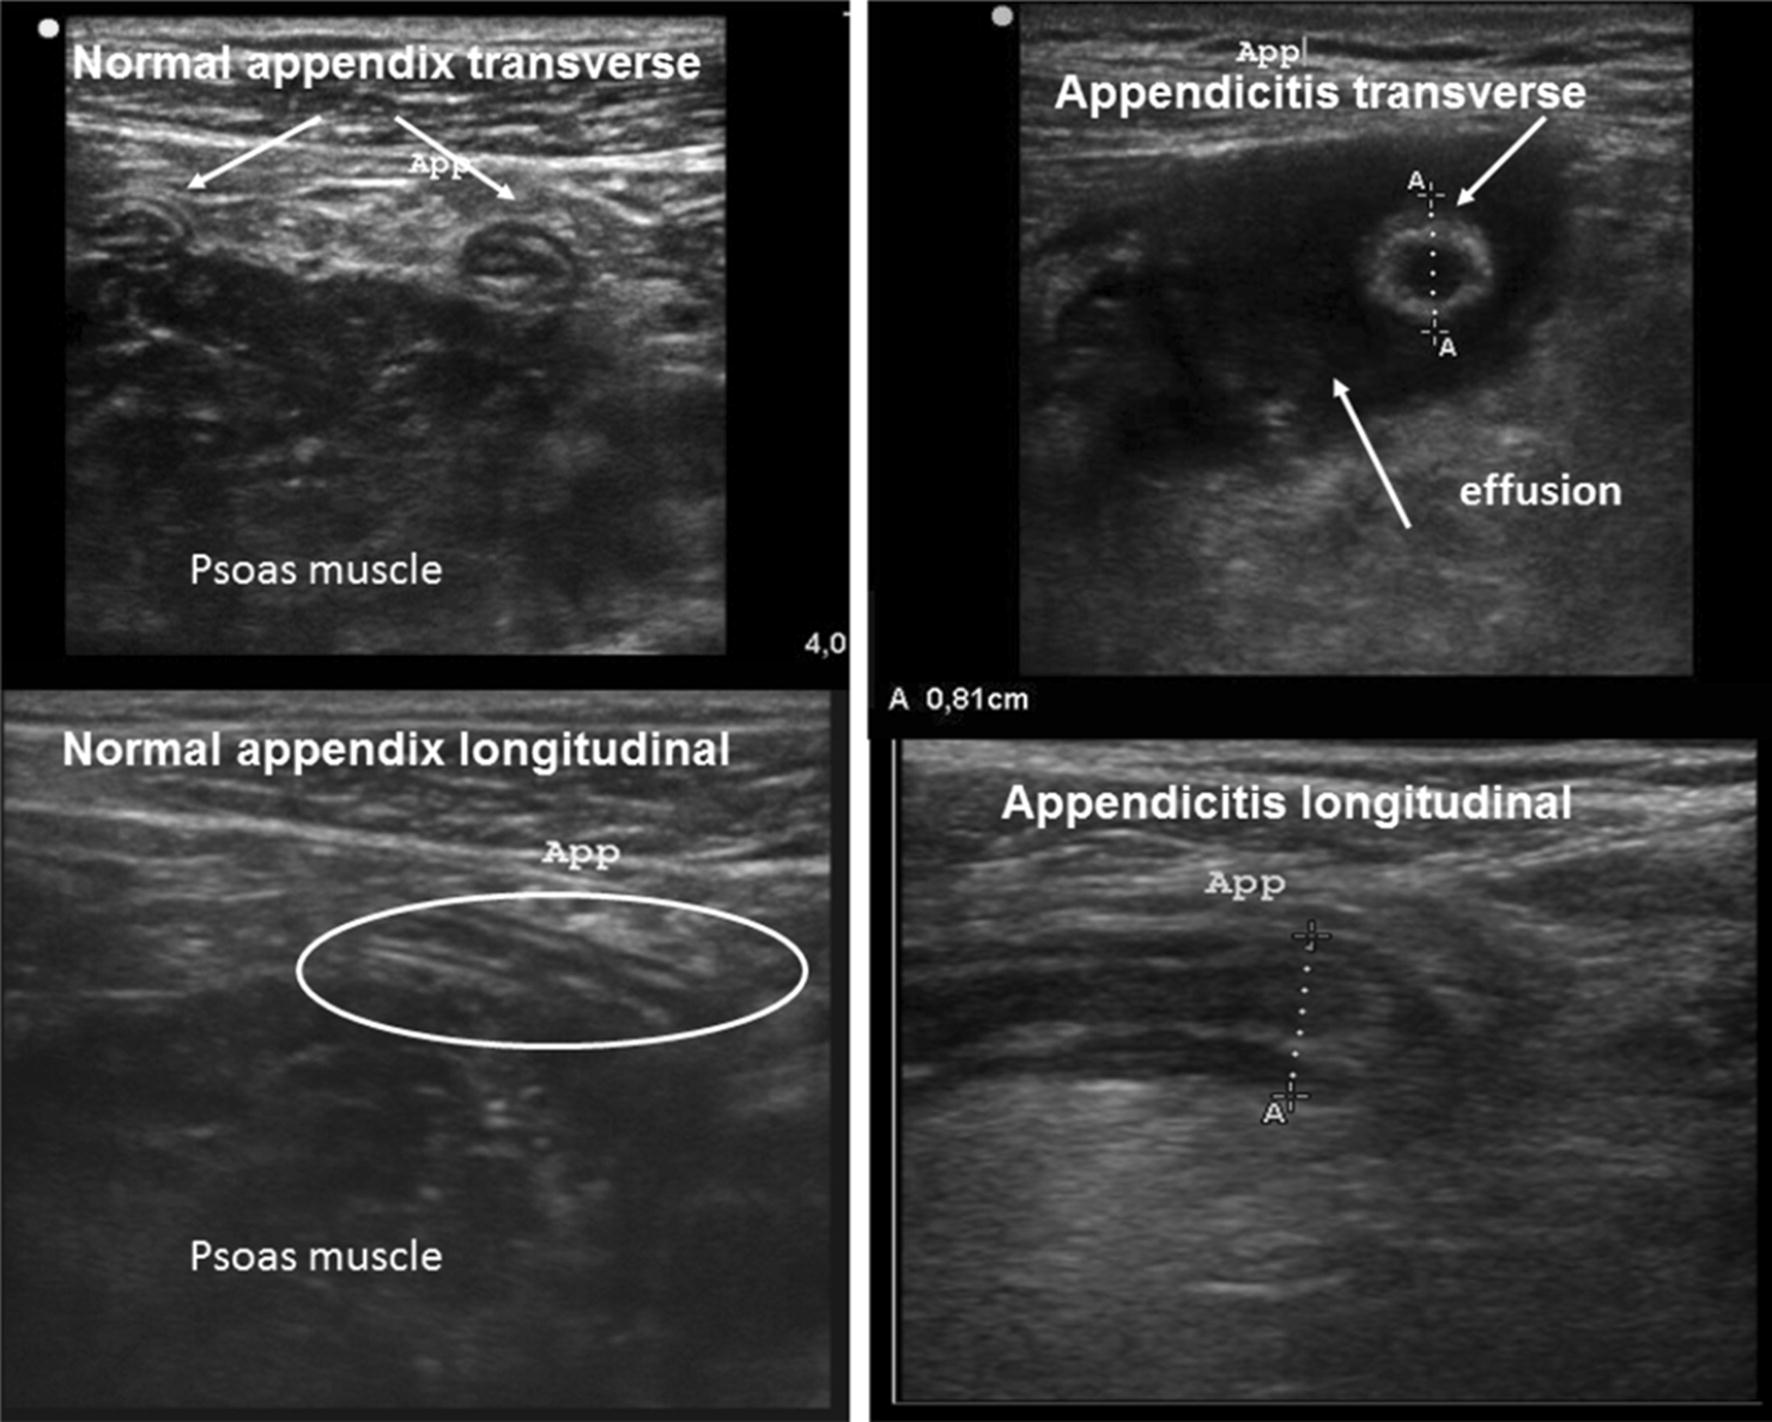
Ultrasound appearance of a normal appendix and a pathological one
